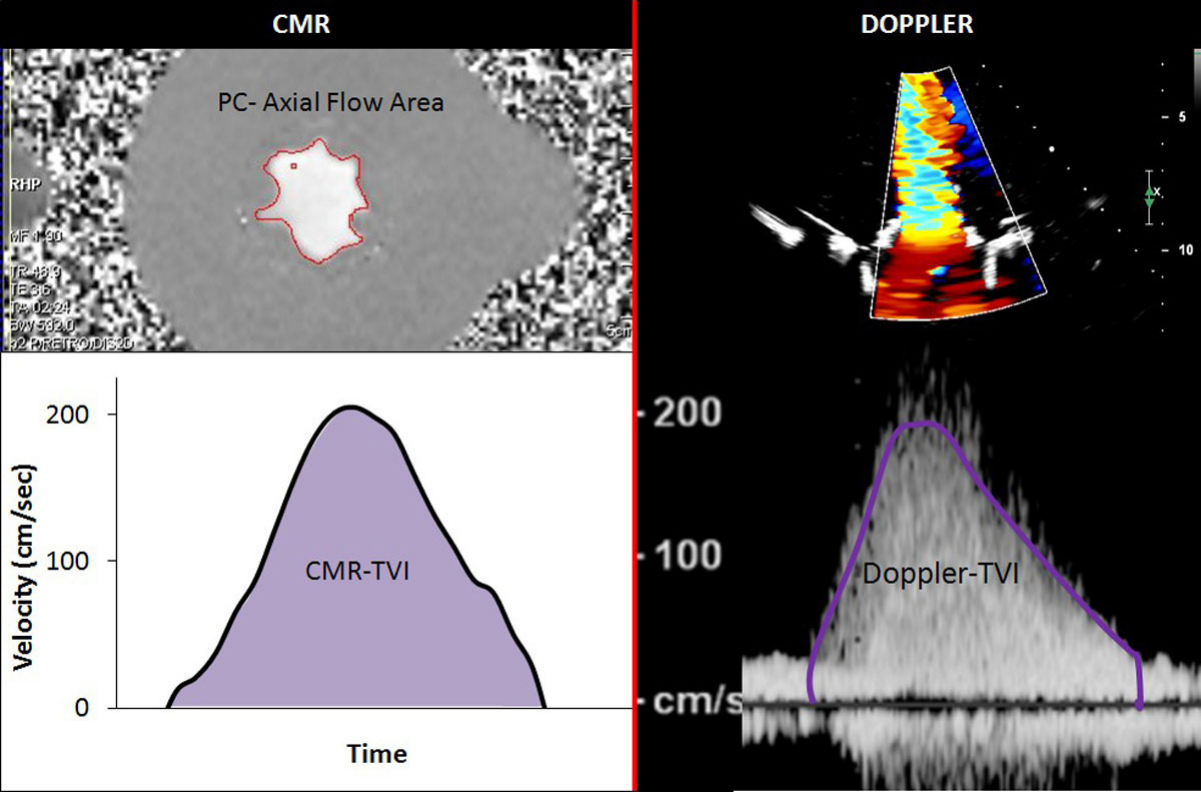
# Bioprosthetic mitral valve effective orifice area by phase-contrast CMR. An in vitro comparison with Doppler echocardiography
